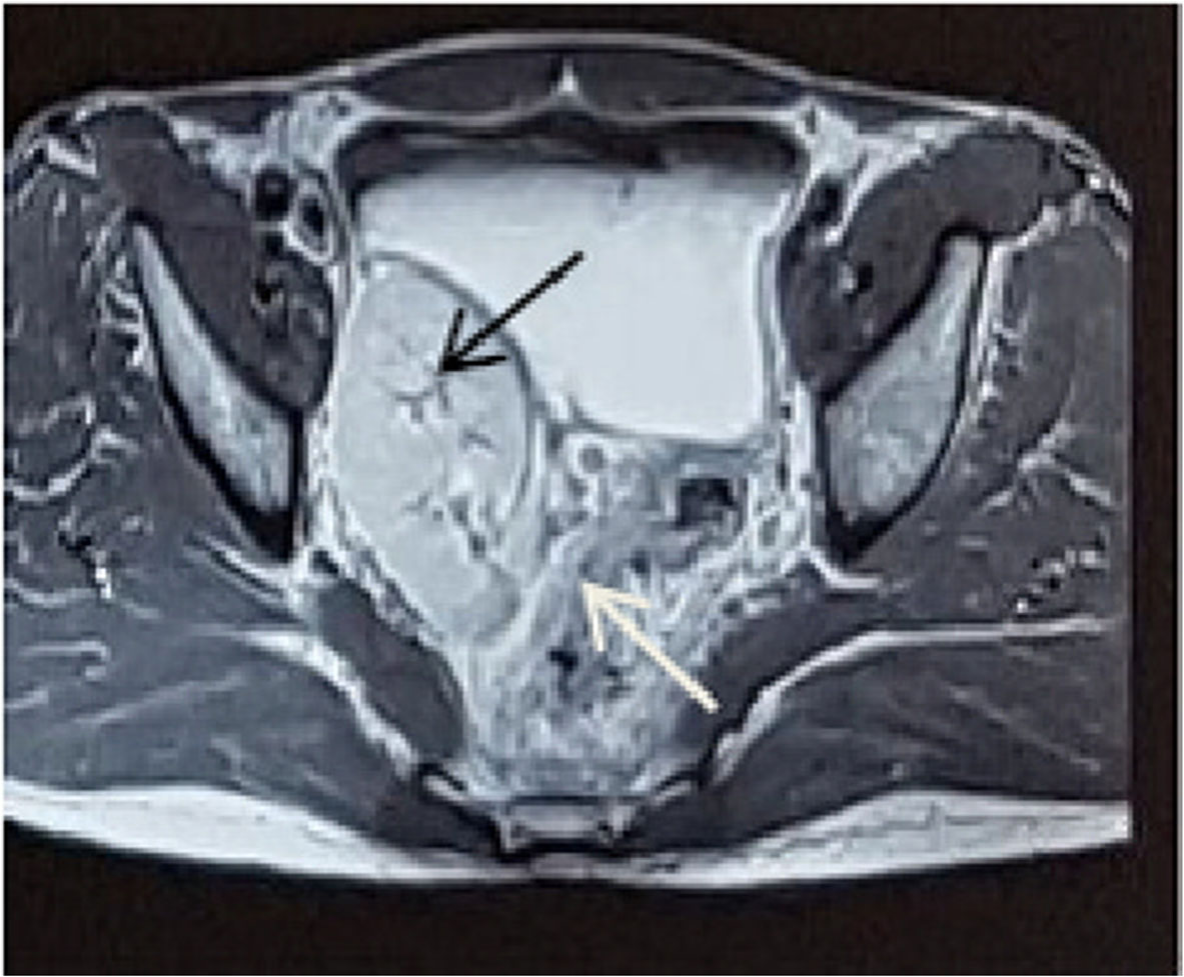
Pelvic-abdominal MRI showing the right kidney in the iliac fossa. Axial view (Black arrow is right pelvic kidney and white arrow is rectal tumor)
